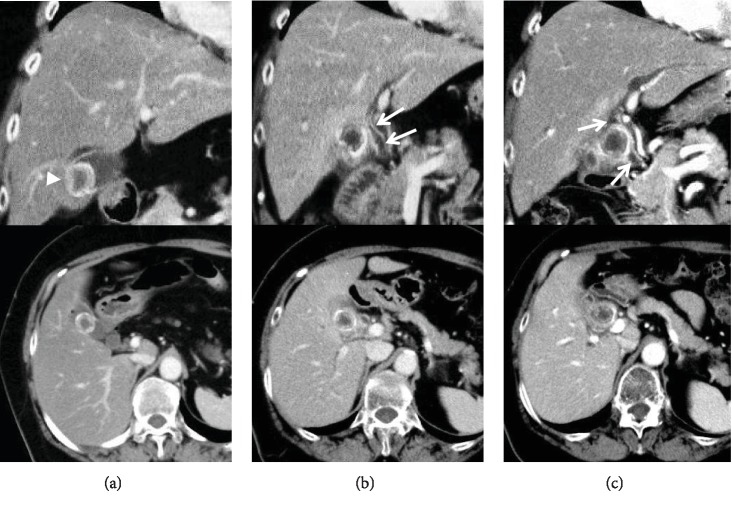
Abdominal CT scan (axial and coronal views): (a) 4 years ago, (b) 6 months ago, and (c) 2 months ago. The CT scan shows a 1.9 cm radiopaque stone (arrowhead in (a)). The gallbladder stone was compressing the common hepatic duct (arrows in (b)). The gallbladder stone had progressively compressed the common hepatic duct (arrows in (c)) in comparison with the situation 6 months previously.
